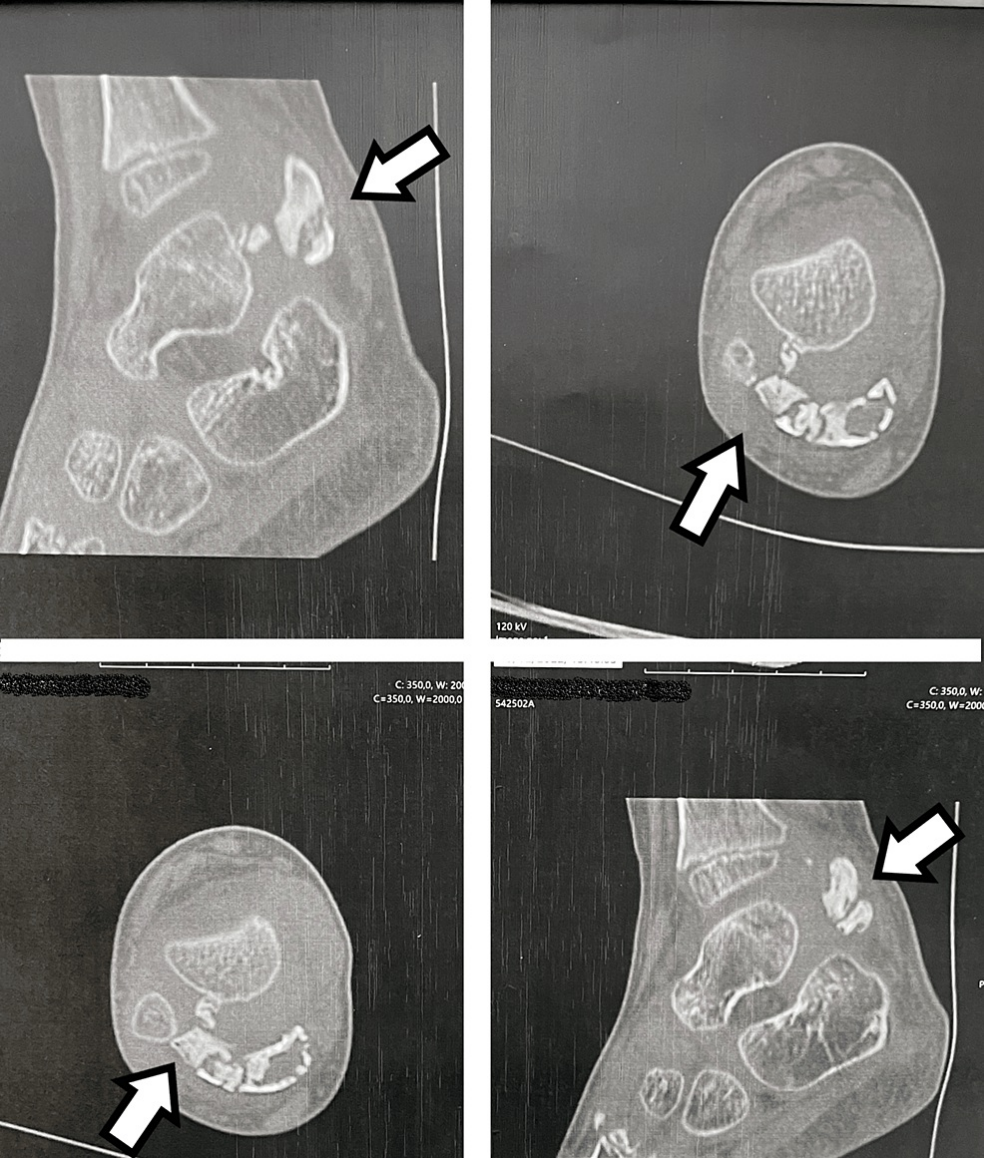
CT scan of the right talus with multiplanar reconstruction. There is extensive calcification of the mass. A small calcified stalk is observed between the lesion and the talus, but no clear continuity of the stalk with the talus is confirmed.
